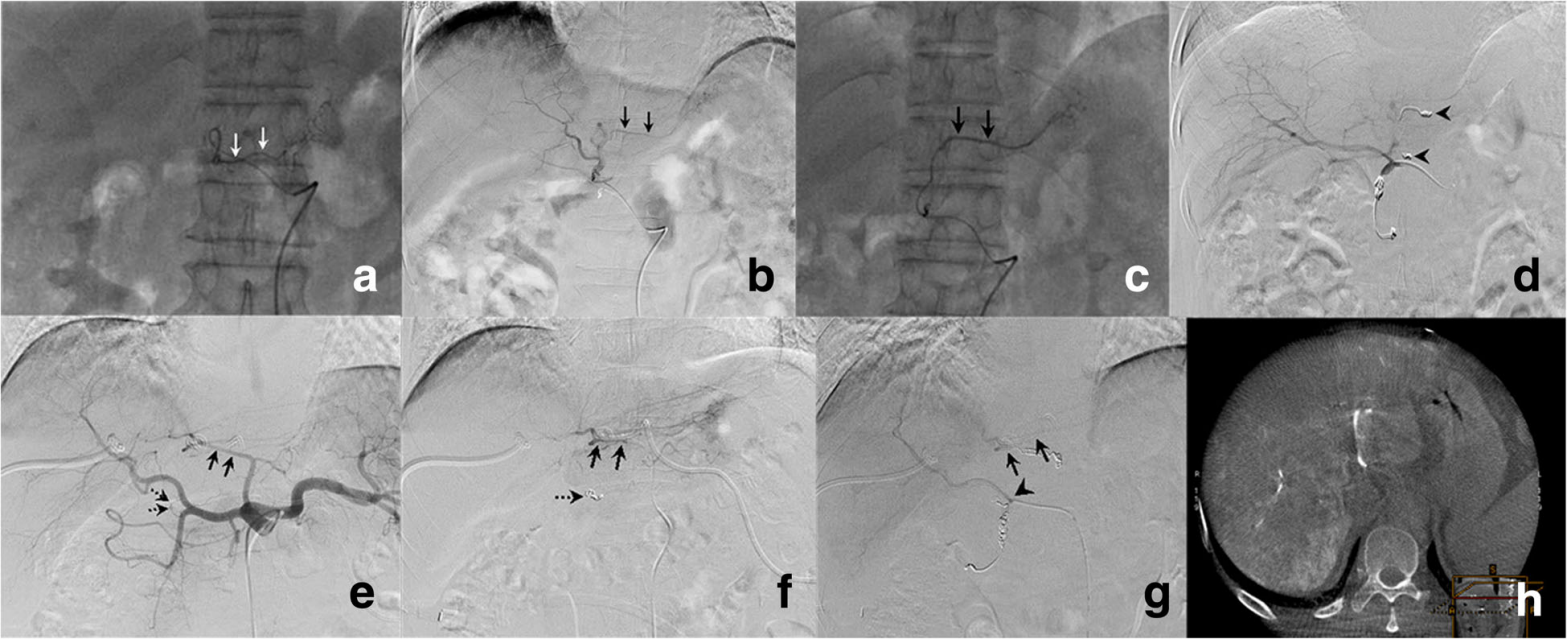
Embolization of extrahepatic arteries and Hepatic artery redistribution embolization. a. Selective right gastric arteriogram from left hepatic artery shows right gastric artery (white arrows), embolized with microcoils; b. Left hepatic arteriogram showed accessory left gastric artery (black arrows) arising from left hepatic artery; c. Accessory left gastric artery (black arrows) was selectively catheterized and embolized with coils; d. Proper hepatic arteriogram shows whole hepatic artery without extrahepatic supplies. Black arrowheads refer to coils embolized in the accessory left gastric artery and right gastric artery. e. Celiac arteriogram indicates replaced left hepatic artery (black arrows) arising from left gastric artery. Black dotted arrows indicate right gastric artery. f. Replaced left hepatic artery (black arrows) selectively catheterized and embolized with microcoils. Right gastric artery was embolized with microcoils (black dotted arrow). g. Proper hepatic arteriogram from side hole (black arrowhead) of indwelling catheter shows whole hepatic arterial flow including right hepatic artery and the redistributed left hepatic artery (black arrows). h. CBCT-proper hepatic arteriogram shows contrast enhancement of the entire liver
